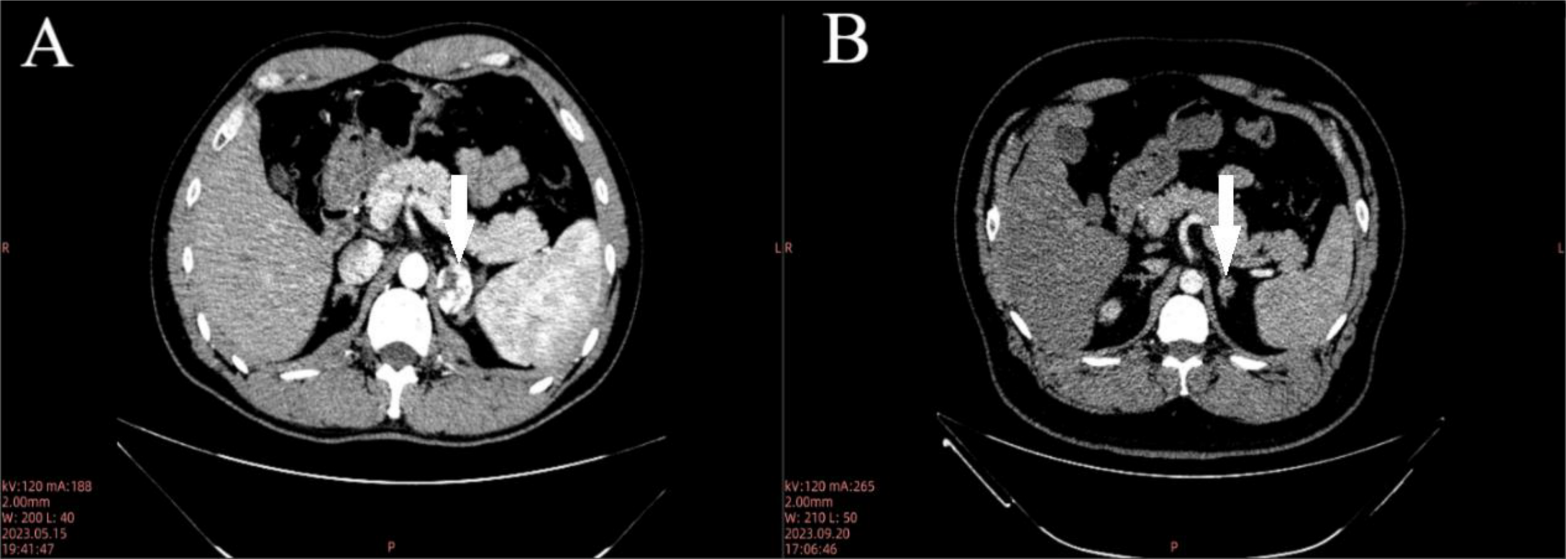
Enhanced CT images of Case 1 (A) and Case 2 (B).
